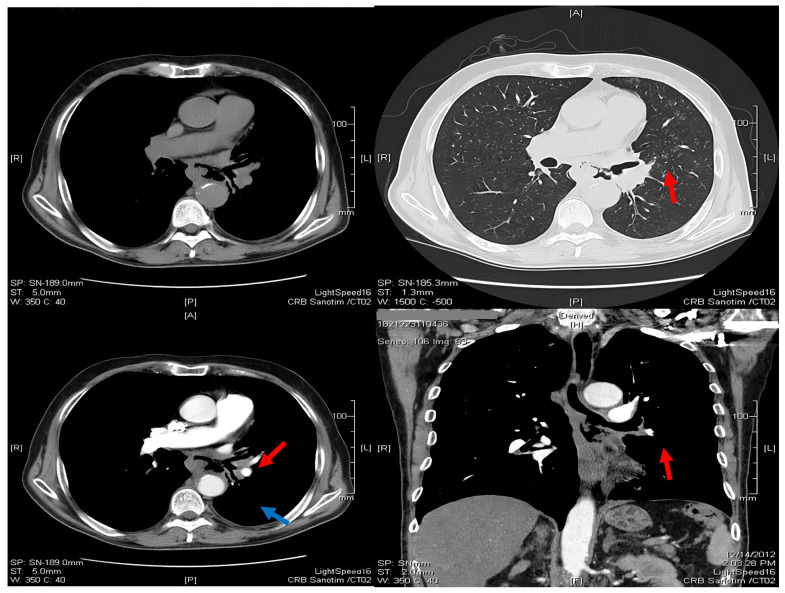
Axial and coronal CT sections showing the BEF between esophageal lumen and left main bronchus (red arrow) and the dense nodule (blue arrow).
